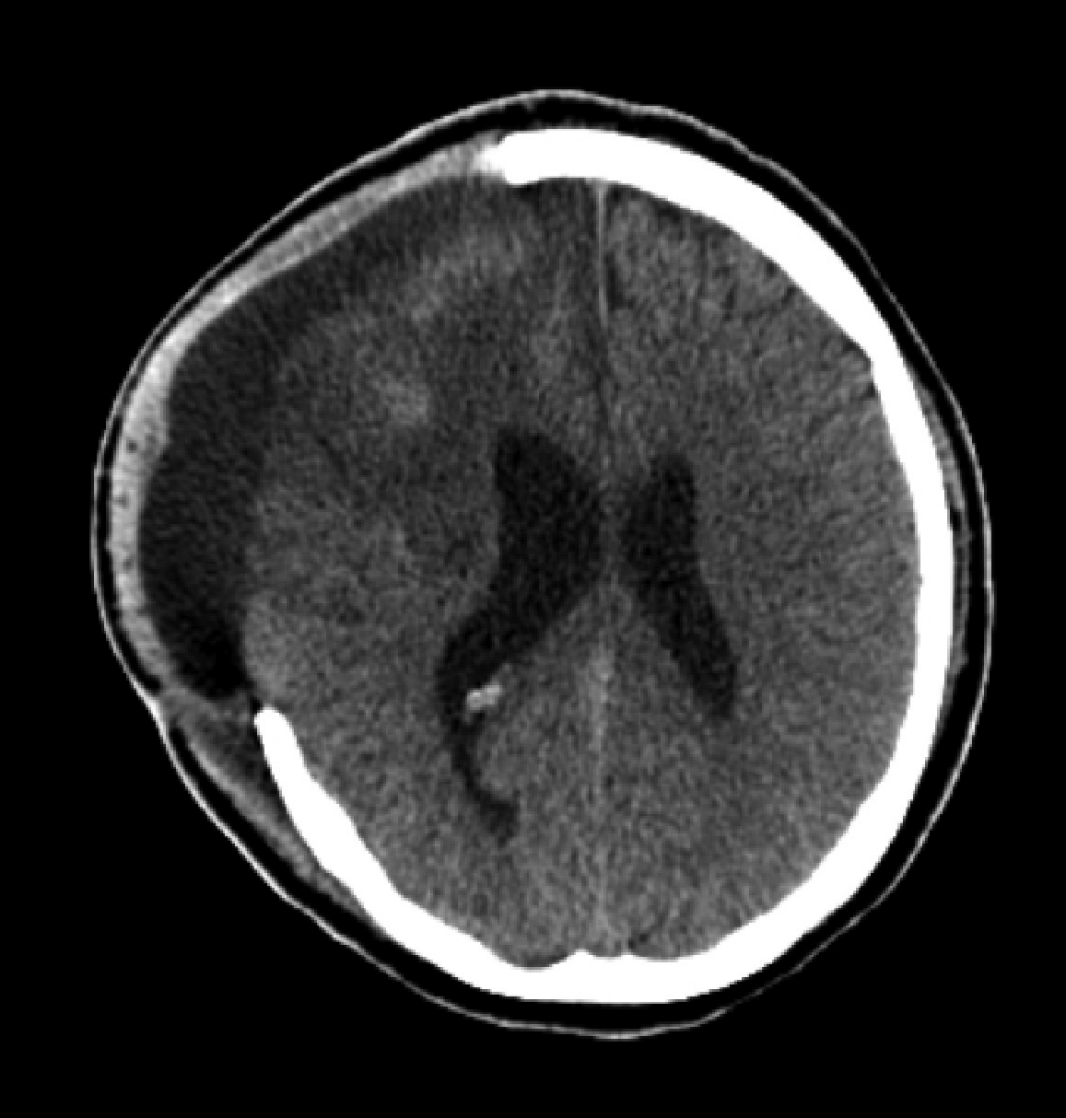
Brain CT scan of the patient shows hypodensities in the right frontal, temporal, and parietal lobes and evidence of craniectomy due to a recent CVA. There was no sign of bleeding, permitting judicious anticoagulation therapy.
